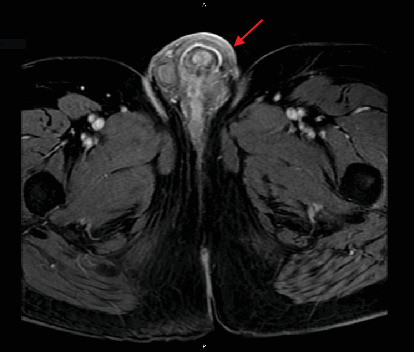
Magnetic resonance imaging (MRI) of the pelvis showing a heterogeneously enhancing lesion measuring 3.7 by 3.6 cm near the left penile base (indicated by the red arrow).
